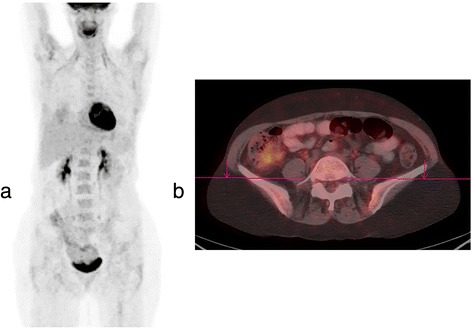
PET/CT of a 54-year-old ovarian cancer patient status post debulking and chemotherapy with no evidence of active residual disease. Concurrent CA-125 was 5. a PET MIP image shows physiologic FDG uptake without evidence of active residual disease. b Axial-fused PET/CT image shows physiologic intestinal FDG uptake in the *right lower quadrant* and the pelvis.
